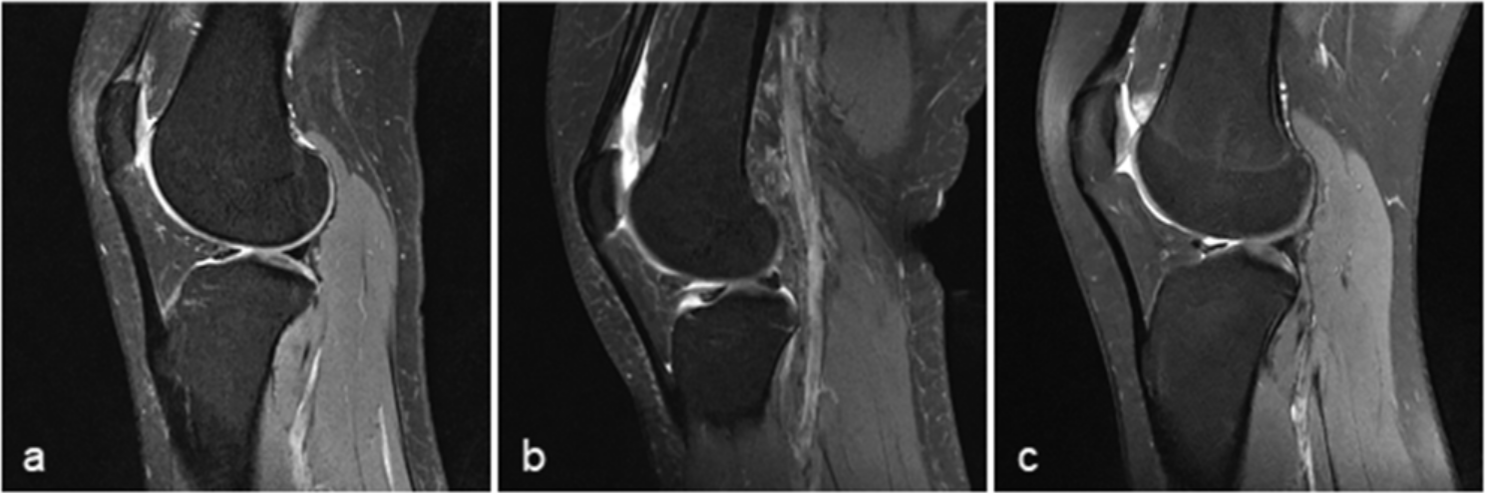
Prefemoral fat pad edema (PFPE). Sagittal magnetic resonance imaging of the knee shows the PFPE scoring system for a normal; b mild or intermediate, grade 1; and c severe or grade 2 PFPE.
